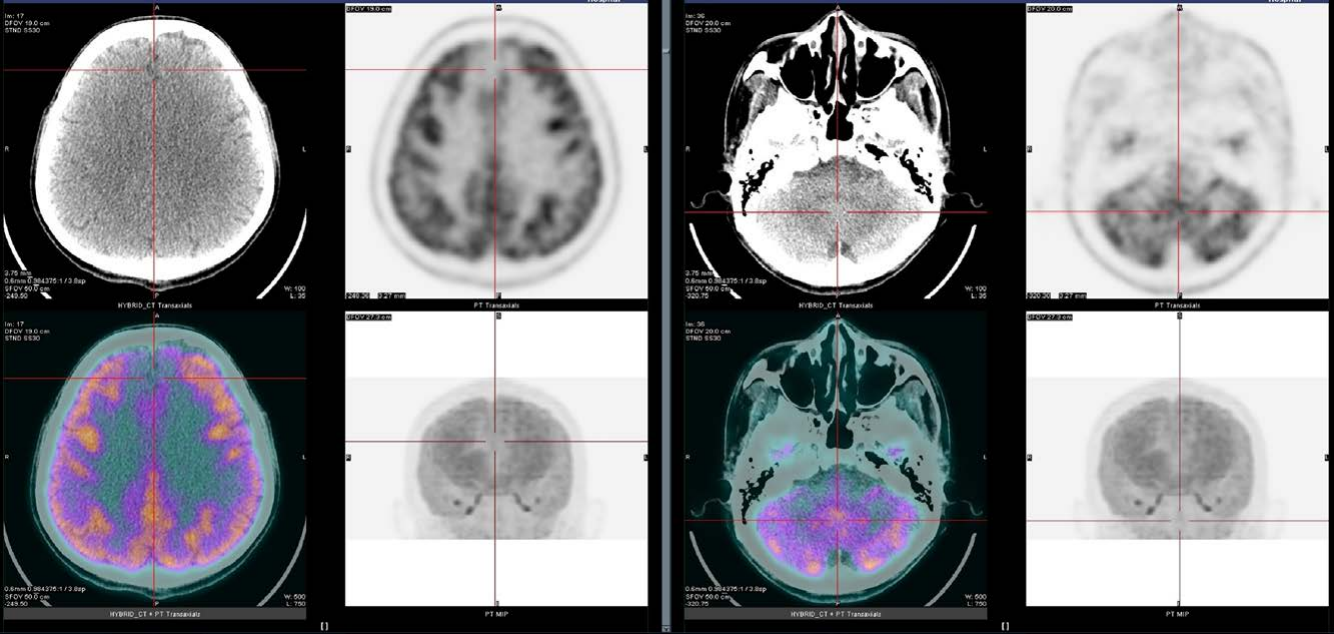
Half a yr later, the follow-up PET/CT showed hypometabolism in the bilateral medial frontal gyrus but no abnormal uptake of FDG in the bilateral cerebellar cortex and dentate nucleus. FDG = fluorodeoxyglucose, PET/CT = positron emission tomography/computed tomography.
